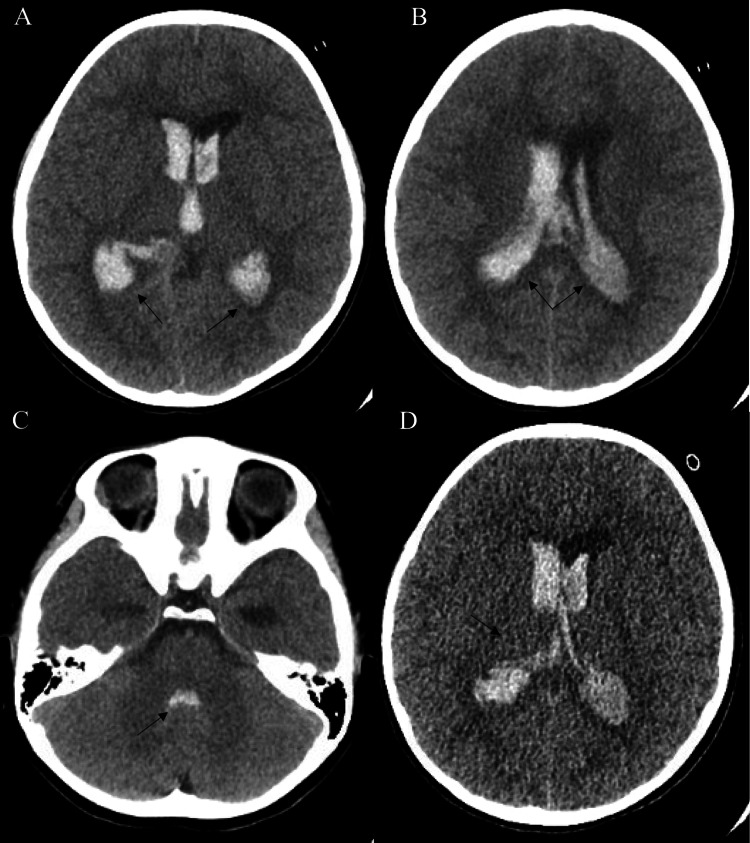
Computed tomography (CT) head without contrast (A) Extensive hemorrhage in the ventricular system with interval dilatation of the temporal horns (black arrows) of both right and left lateral ventricles. (B) Extensive hemorrhage in the lateral ventricles (black arrows). (C) Hemorrhage in the fourth ventricle (black arrow). (D) Hemorrhage likely originating from the right thalamus (black arrow).
